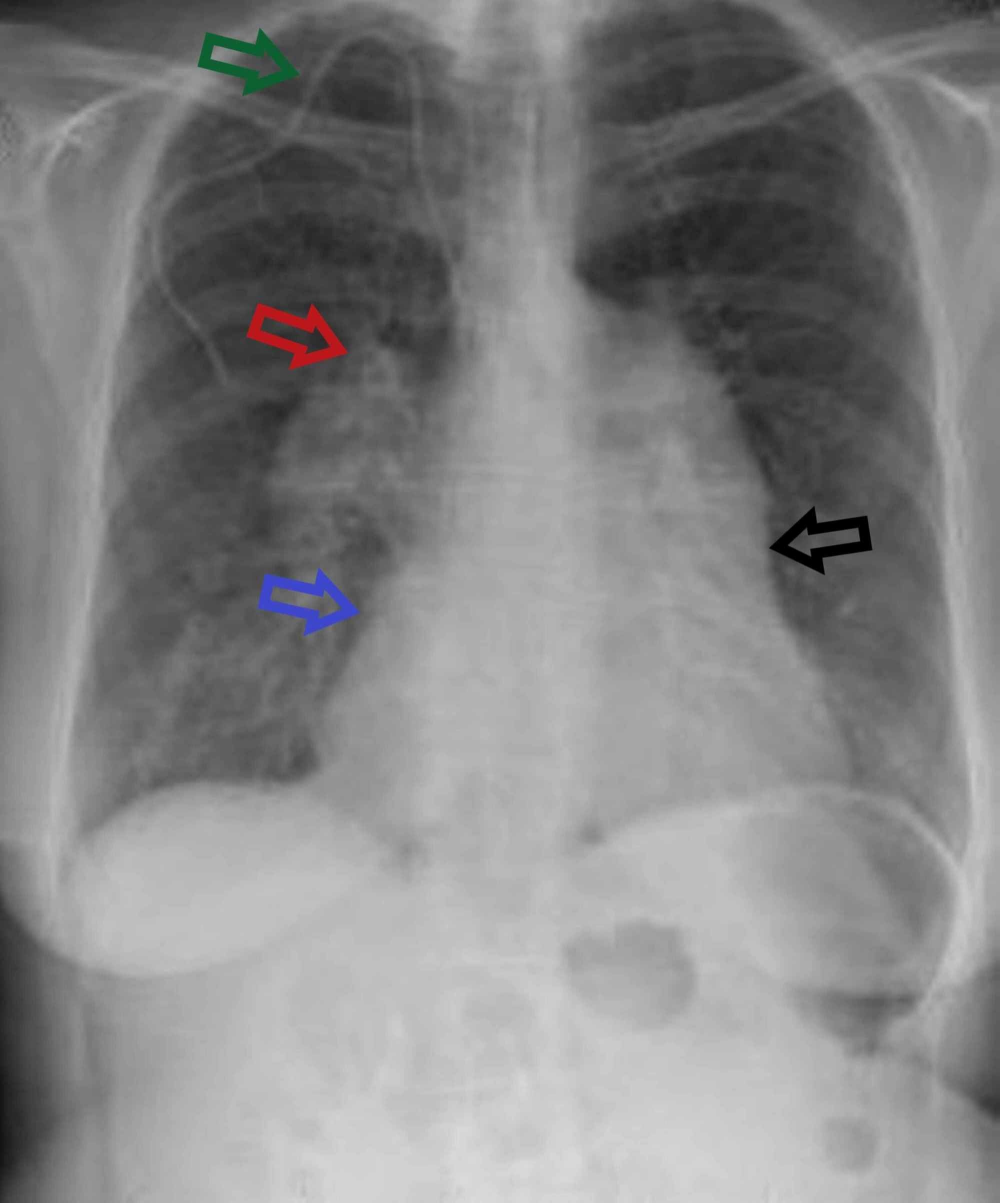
Chest X-ray of a patient with pulmonary hypertension The X-ray depicts cardiomegaly (black arrow), increased right ventricular diameter (blue arrow), pulmonary congestion (red arrow), and central venous access (green arrow)
